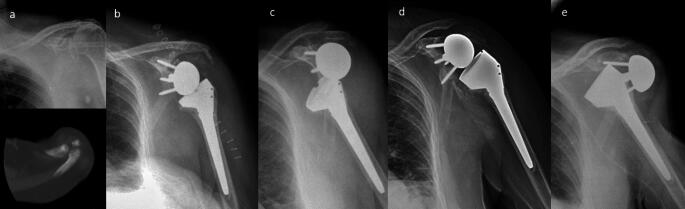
A 72-year-old woman with recurrent prosthetic instability (a) X-ray and axial CT plane showing rotator cuff arthropathy with os acromiale (preacromion). (b) Immediate postoperative radiographic control. (c) Prosthetic dislocation after two years. (d) Radiographic control after revision surgery. (e) Third dislocation, no further revision surgery performed
